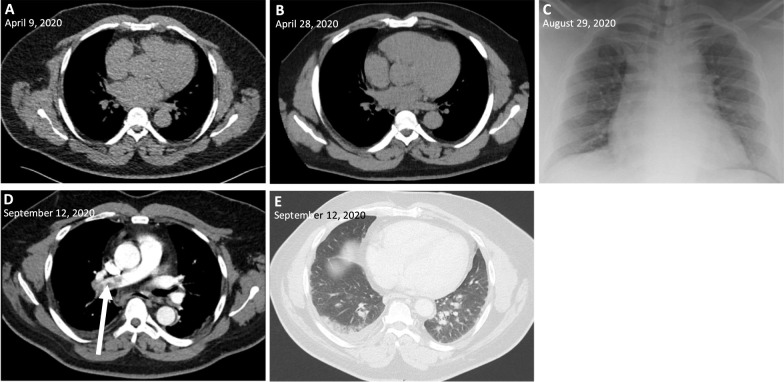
Chest computed tomography and radiograph images during the disease course. A–B, chest computed tomography (CT) without abnormalities; C bedside chest radiograph without alveolar consolidations; D CT pulmonary angiogram showing pulmonary embolism (arrow); E chest CT showing peripheral ground-glass opacities and pleural effusion in the right lower lobe
